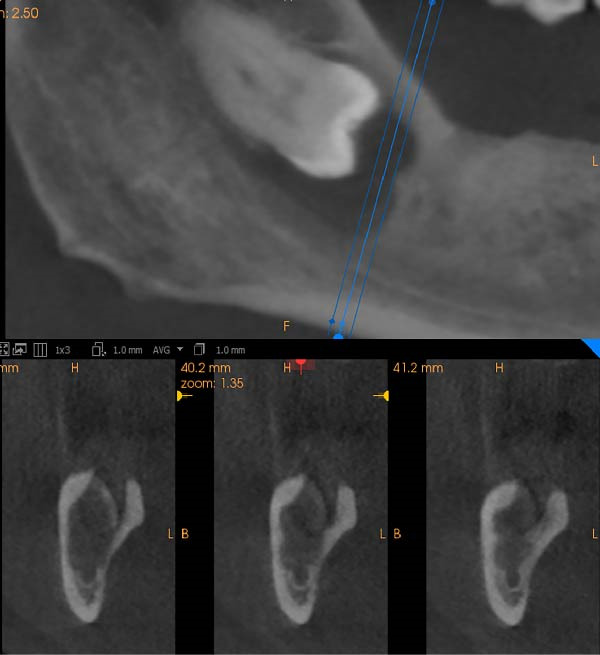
(Top) Cropped, reformatted panoramic image showing a pericoronal radiolucency associated with the impacted right mandibular third molar. (Bottom) Buccolingual CBCT cross‐sections demonstrate a well‐defined lesion with scalloped borders and no cortical expansion.
